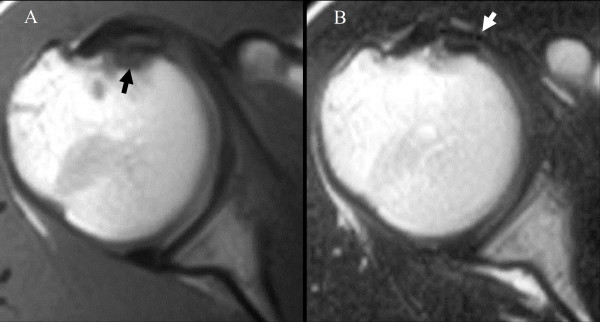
A, T1-weighted image showing a low-intensity area at the lesser tuberosity (black arrow) B, T2-weighted image showing a high-intensity area of the subscapularis tendon (white arrow).
